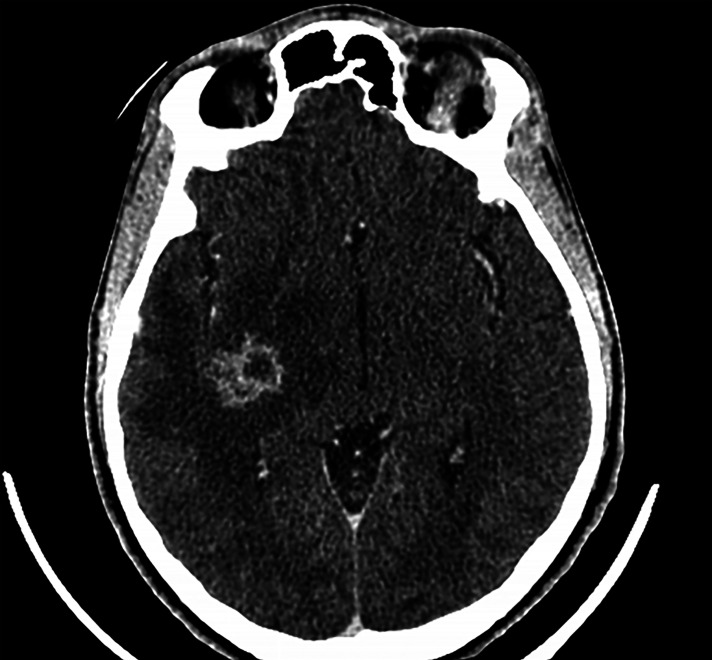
Brain Computed Tomography-scan with nodular, right insular lesion with cocoon enhancement and peri-lesional edema.
